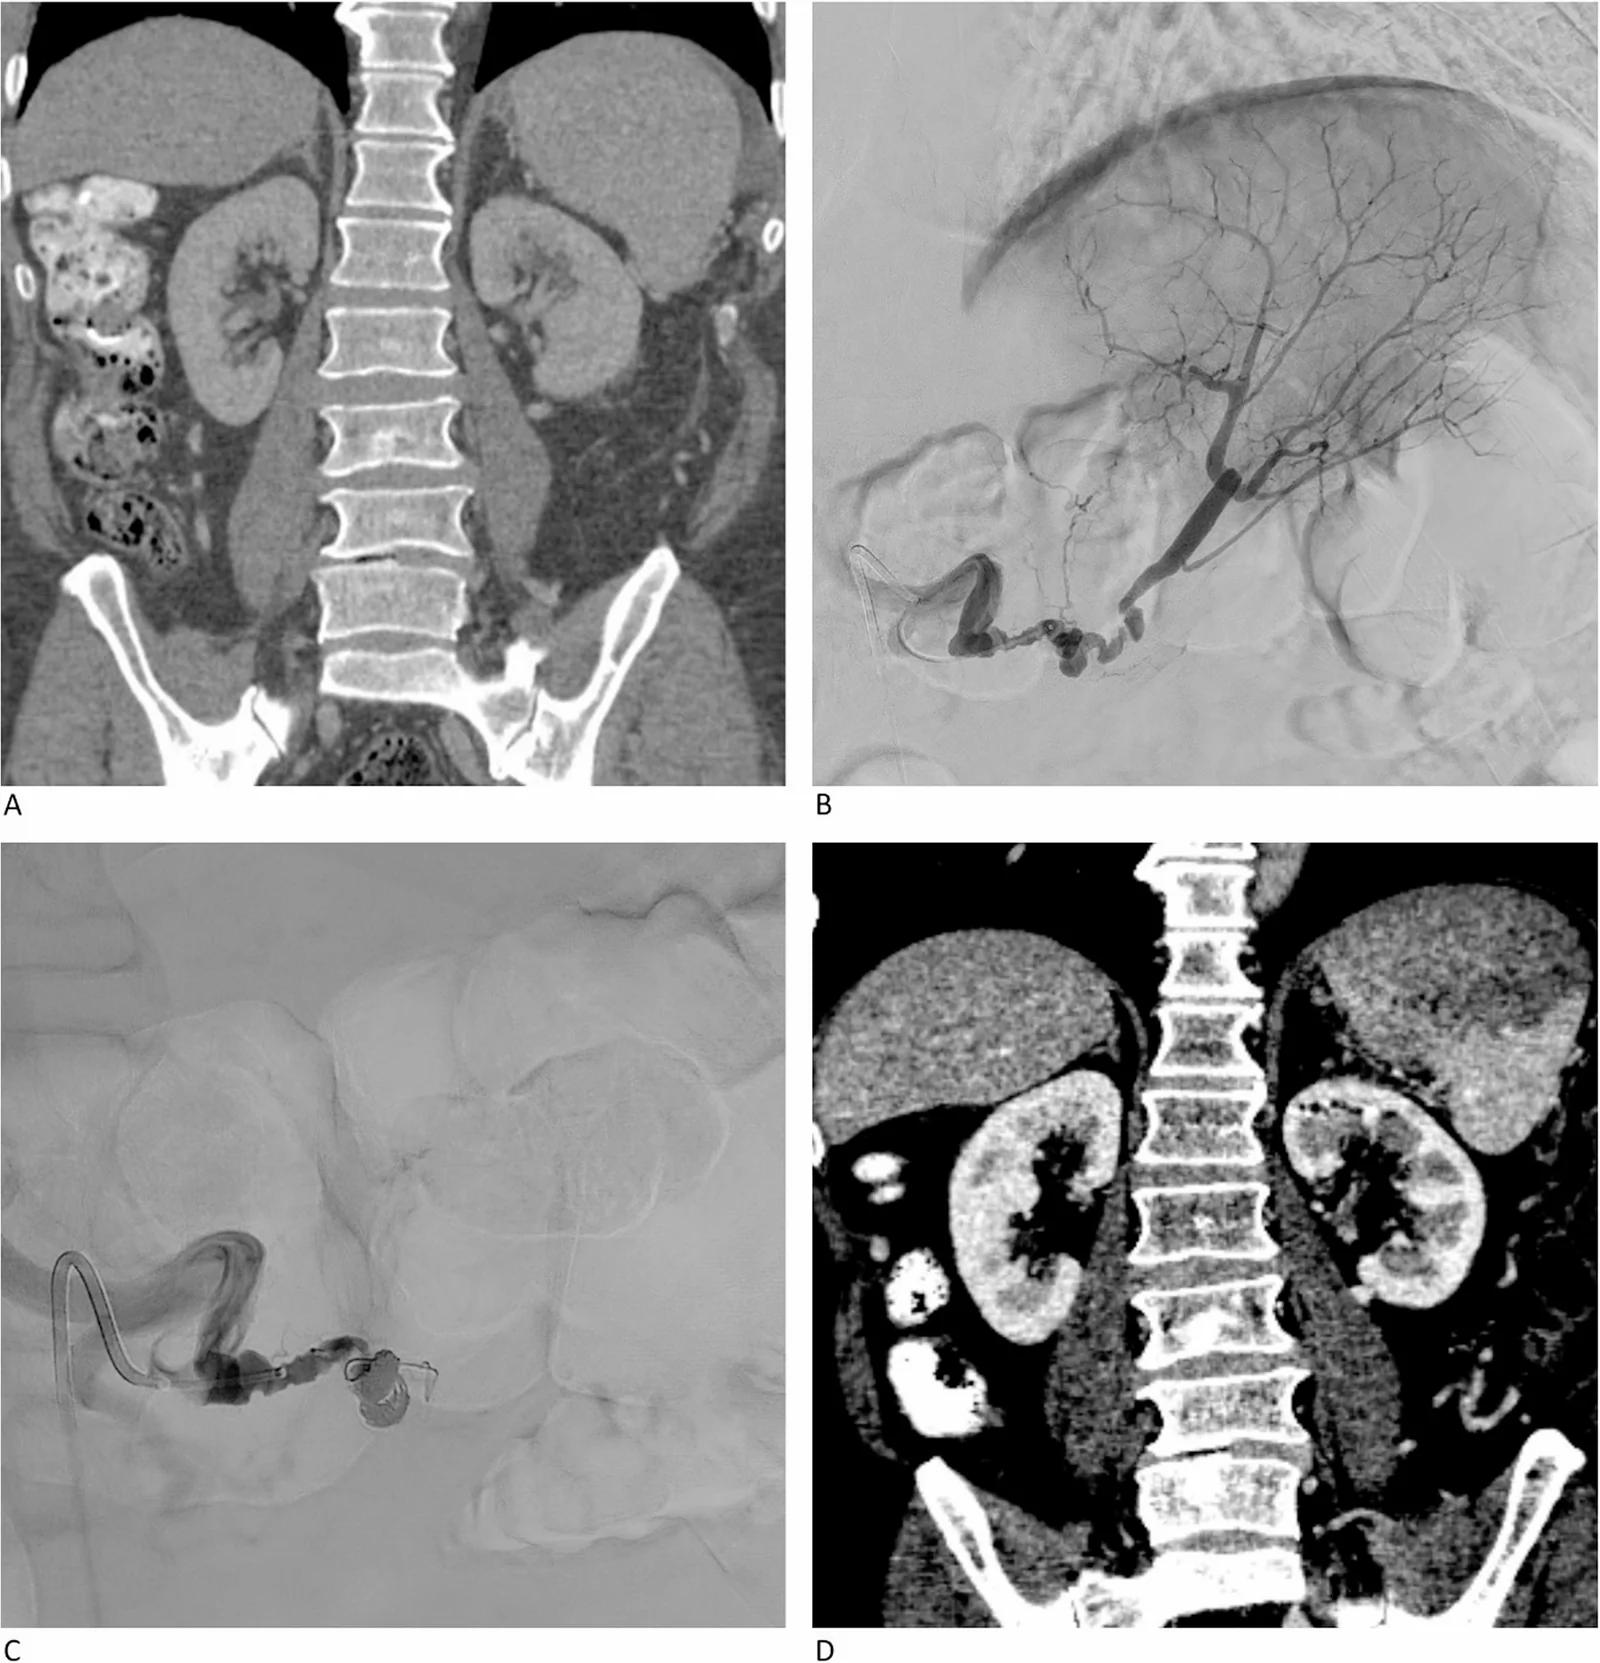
Example case of a 47-year-old man with pancreatic cancer undergoing PSAE. The patient developed CIT under chemotherapy with FOLFIRINOX, with a nadir platelet count of 67 × 10(9)/L. (A) Pre-procedural CT angiogram showing an enlarged spleen (pre-SAE volume 409.1 cm3). (B) Intra-procedural angiogram showing the splenic artery and the enlarged spleen. (C) Intra-procedural angiogram after placement of coils in the proximal splenic artery. (D) Post-procedural CT angiogram at 2-month follow-up showing splenic infarction (post-SAE enhanced volume 126.7 cm3, splenic infarction rate 69.0%). Post-SAE platelet count increased to 190 × 10(9)/L 55 days after the procedure
